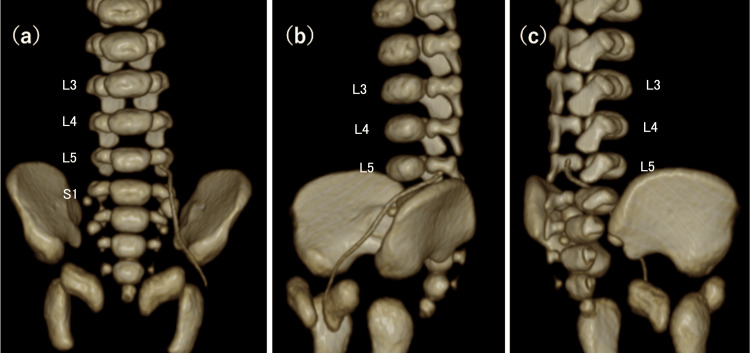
Three-dimensional reconstructed CT These images show the catheter passing from the left ascending lumbar vein through the intervertebral vein into the internal vertebral venous plexus, with the catheter tip located within the spinal canal. Vertebral levels (L3, L4, L5, and S1) are labeled on this image. (a) Anterior view. (b) Left anterolateral view. (c) Right posterolateral view. CT: computed tomography
